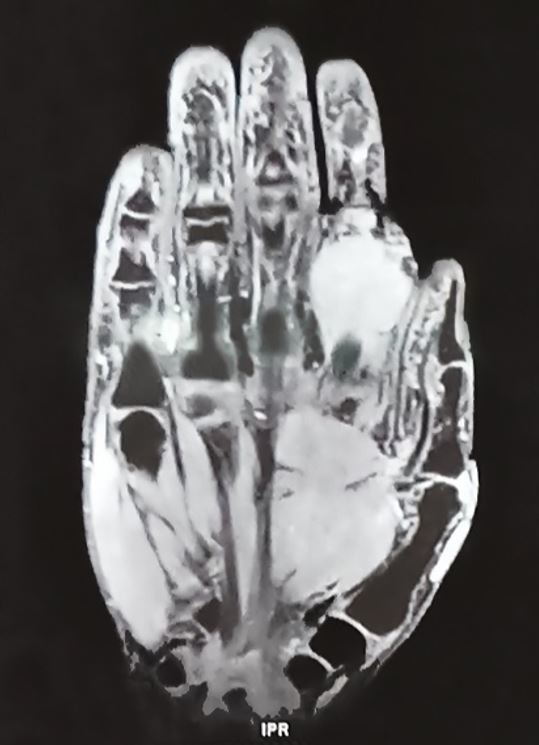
MRI of the right hand, T1 weighted image showing a well-defined multilobulated mass.
